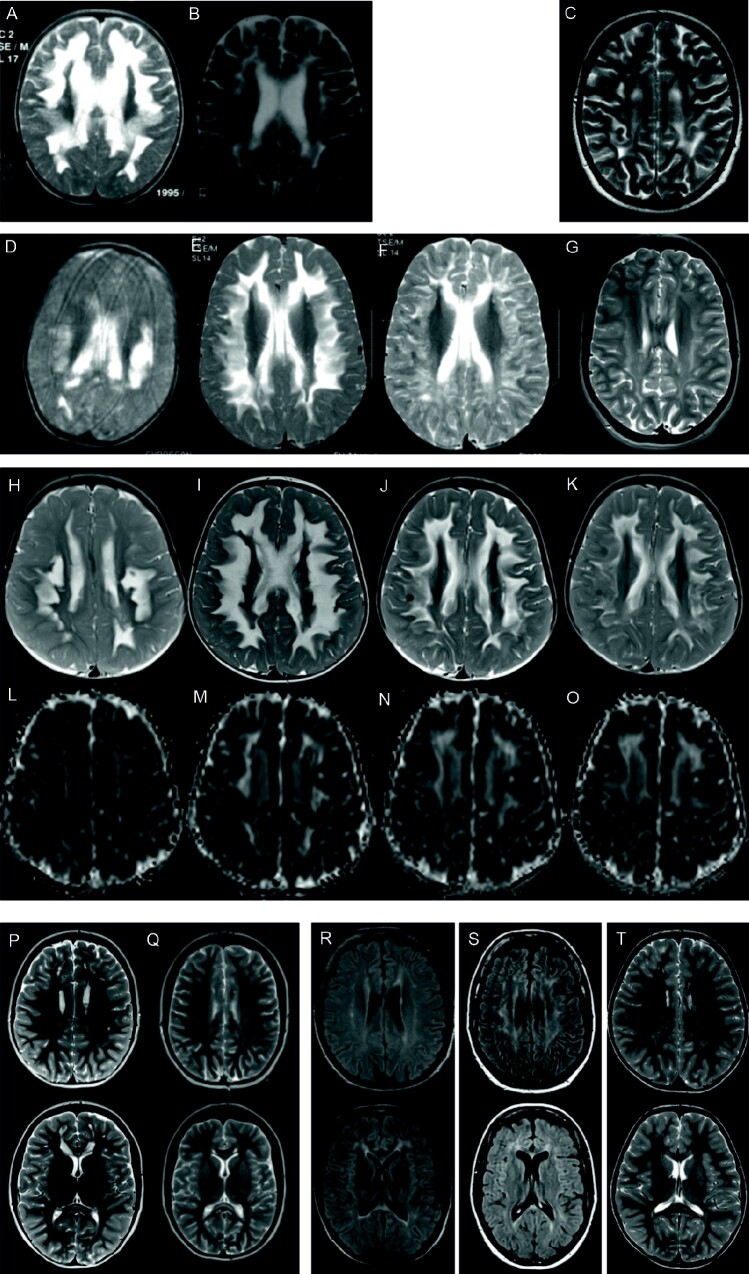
MR imaging of the family members. (A–K) Axial MR images of the patients with the remitting leukodystrophy. (A–C) T2 weighted images of patient III.2 at age 14 months, 22 months and 20 years. (D–G) T2 weighted images of patient III.5 at age 11 months, 21 months, 32 months and 24 years. (H–K) T2 weighted images of patient IV.1 at age 13, 19, 25 and 29 months. (L–O) Apparent diffusion coefficient of patient IV.1 at corresponding time points. (P and Q) T2 weighted images of patient III.4 at age 10 in P and 15 years in Q with residual WM lesions, pronounced frontally and in the genu corpus callosum. (R) Fluid-attenuated inversion recovery (FLAIR) images of patient II.2 at age 38 years revealing frontally pronounced residual WM lesion. (S) FLAIR images of patient II.4 at age 46 years with similar residual pattern and global atrophy. (T) T2 weighted images of patient IV.2 at age 4 years. Of note are the subtle WM hyperintensities in genu and splenium, comparable to patient III.4, II.2, II.4, although no metabolic crisis occurred.
